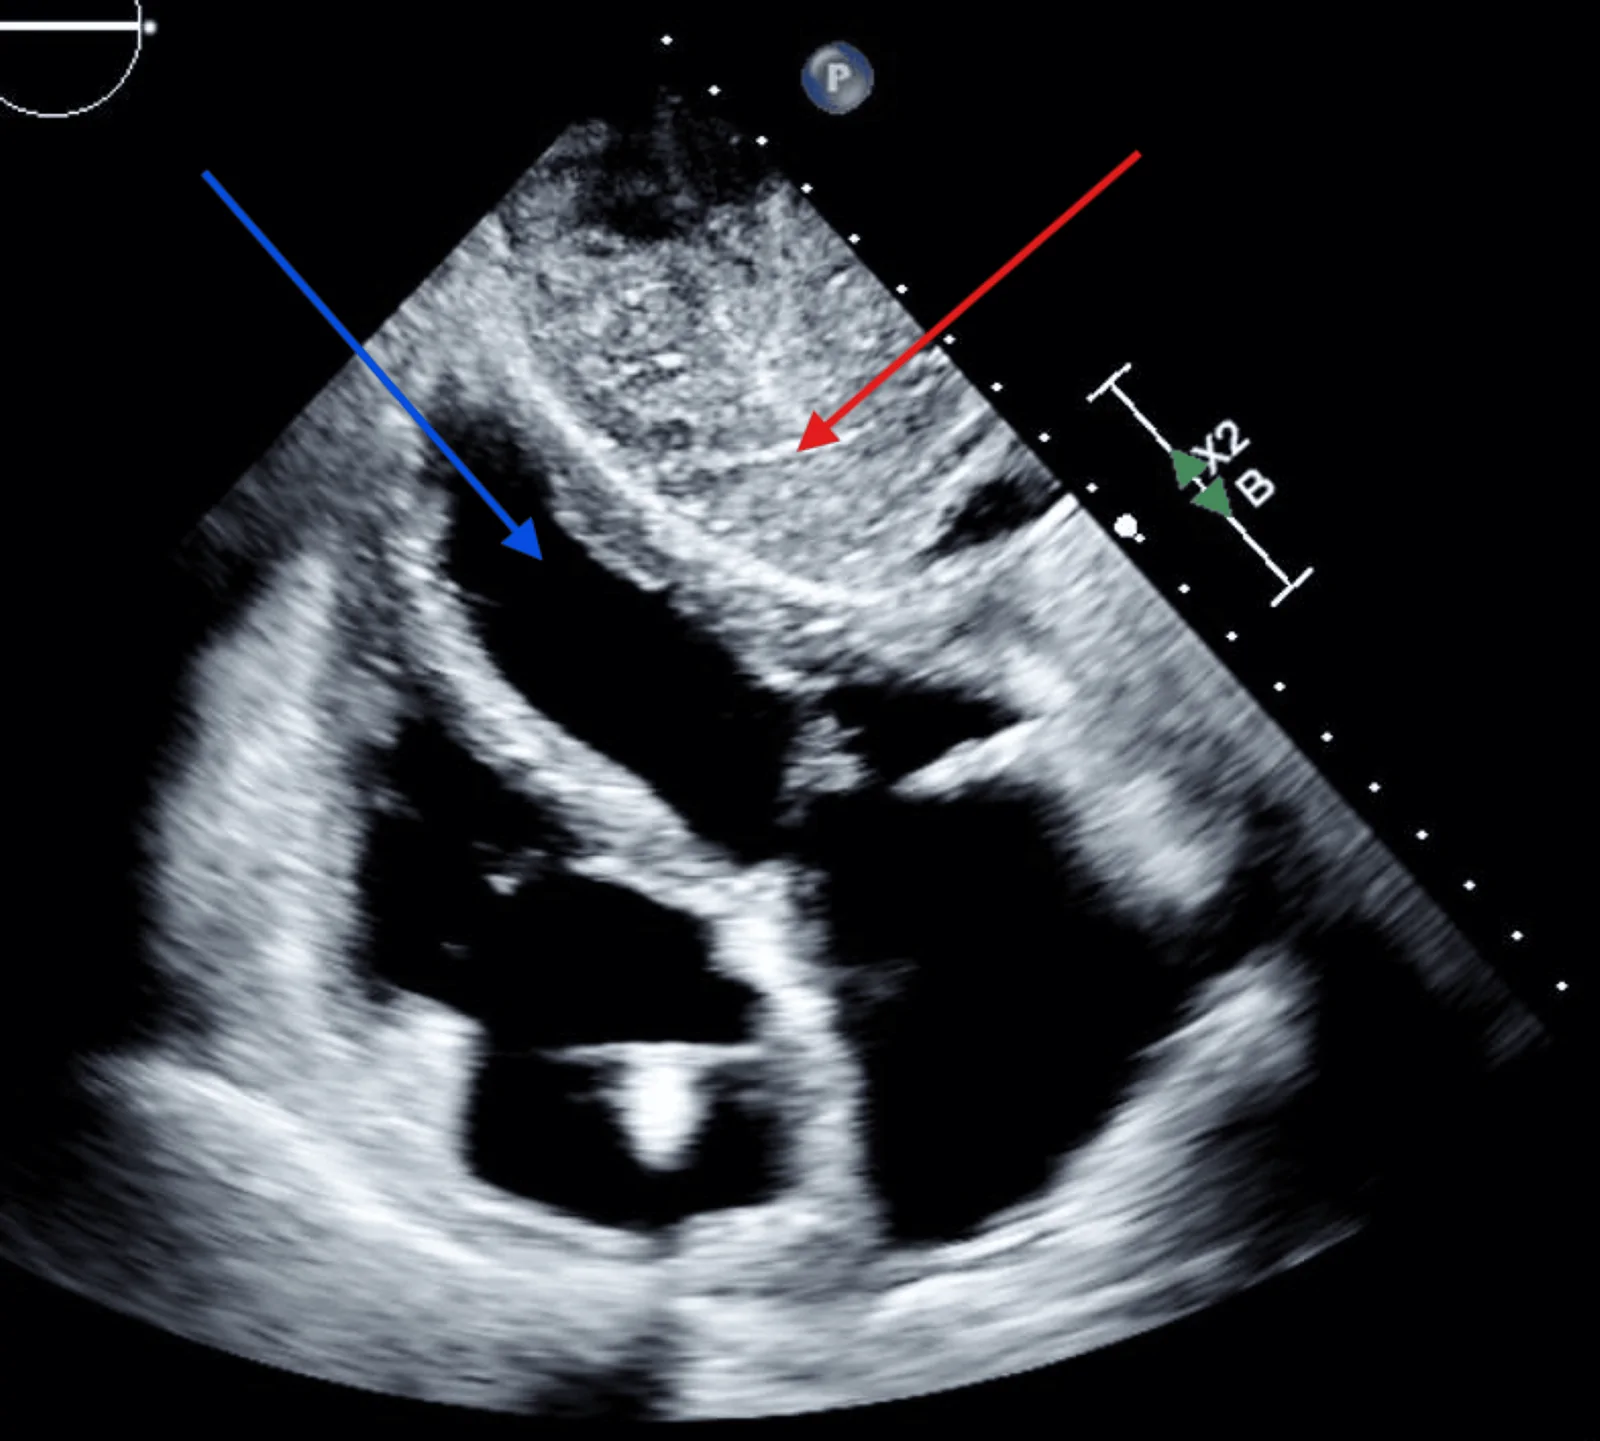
Echocardiogram in diastole showing the left ventricle (blue arrow) and the pleural haematoma (red arrow).
